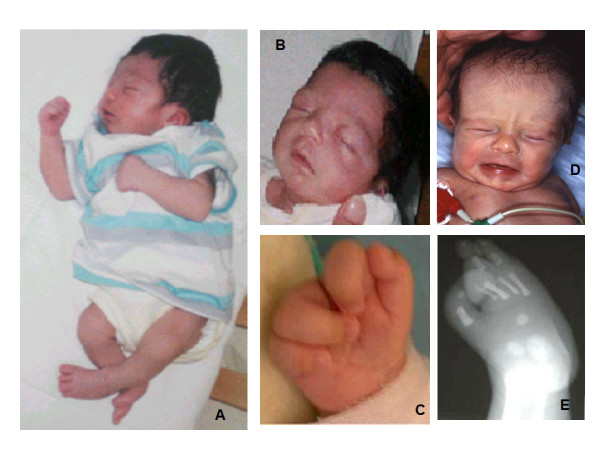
Facial and limb features of two patients with the acro-cardio-facial syndrome: A, B, C, E: patient reported by Mingarelli et al., 2005 [4]; D: patient reported by Giannotti et al., 1995 [2](2a in Table 1). A: Lateral total body view; B: Facial appearance of patient 4, showing high forehead, prominent eyes, long eyelashes, hypertelorism, broad nasal root; C: Ectrodactyly of right hand; D: Facial appearance of patient 2a, showing high forehead, broad nasal root, low-set dysmorphic ears; E: X-ray of right foot, showing split foot malformation with five metatarsal (including a very hypoplastic second metatarsal) and absence of all the phalanges of second and third toes.
